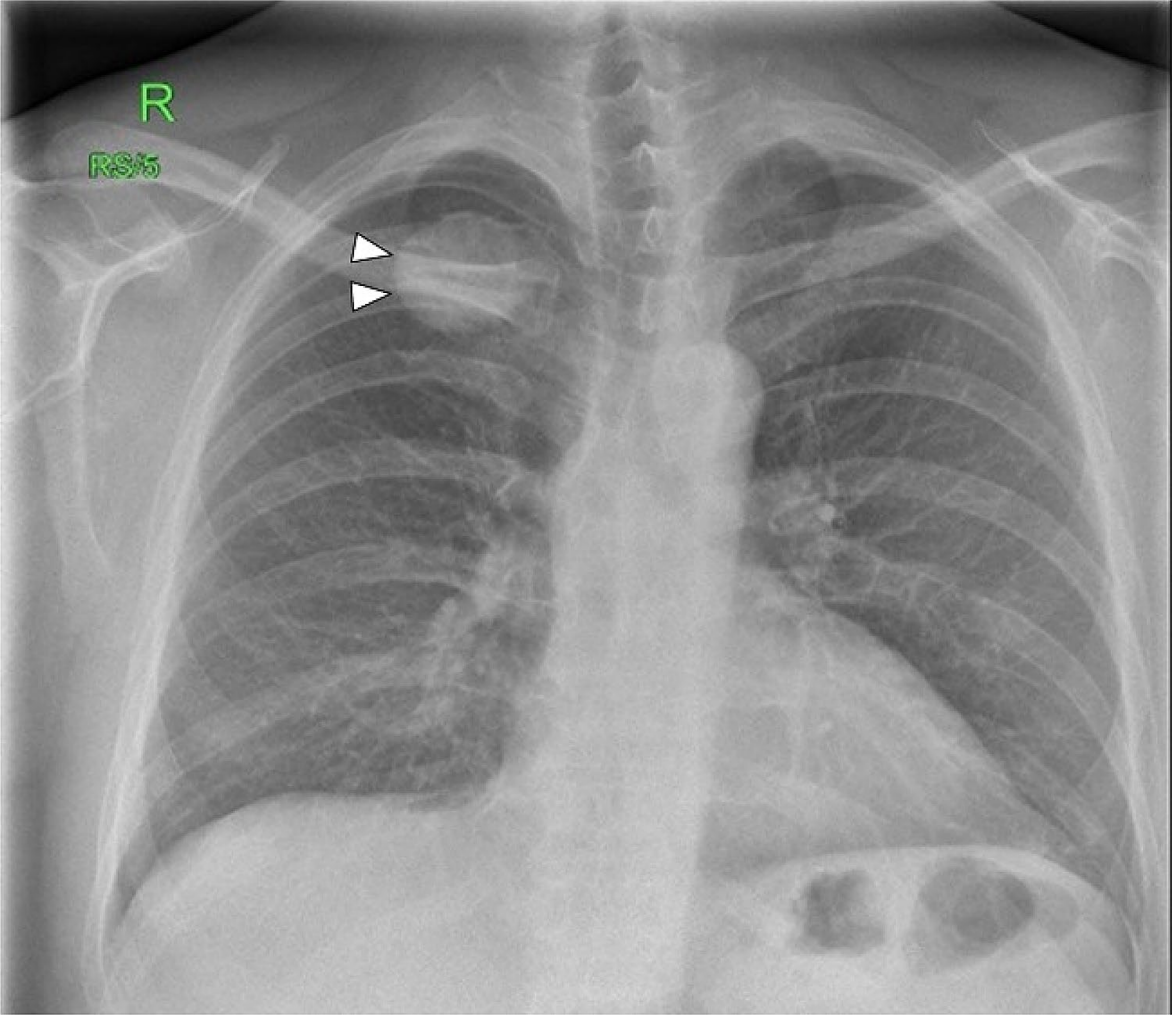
47-year-old man with a PMT of the 4th rib. He presented to the rheumatology service, with an 18-month history of recurrent back pain and unexpected weight loss. On physical examination, he had reduced spinal movement and point tenderness over his sacroiliac joints. Chest X-ray shows a well-defined rounded dense lesion in the 4th rib, with uniform calcification
